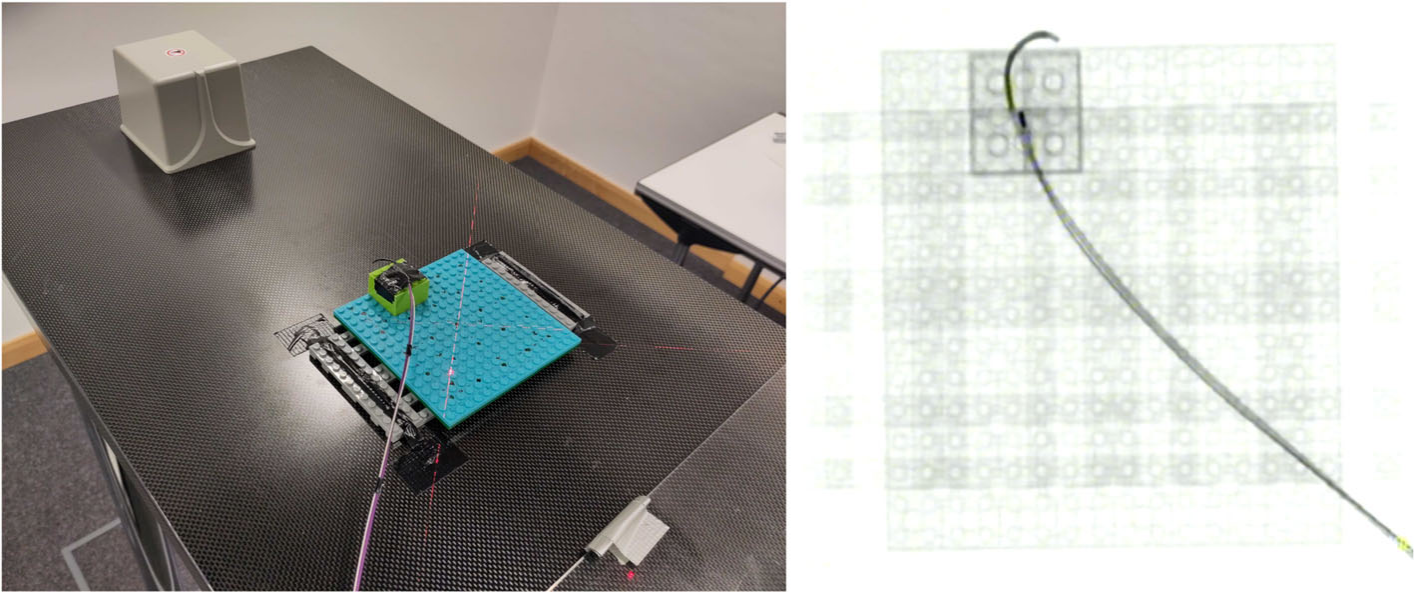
Left: Lego calibration board and sensor fixture for EMT compensation. $$25\times 25$$ training points are marked in a grid, whereas validation points are taken arbitrarily. Right: Same Lego board under X-ray
